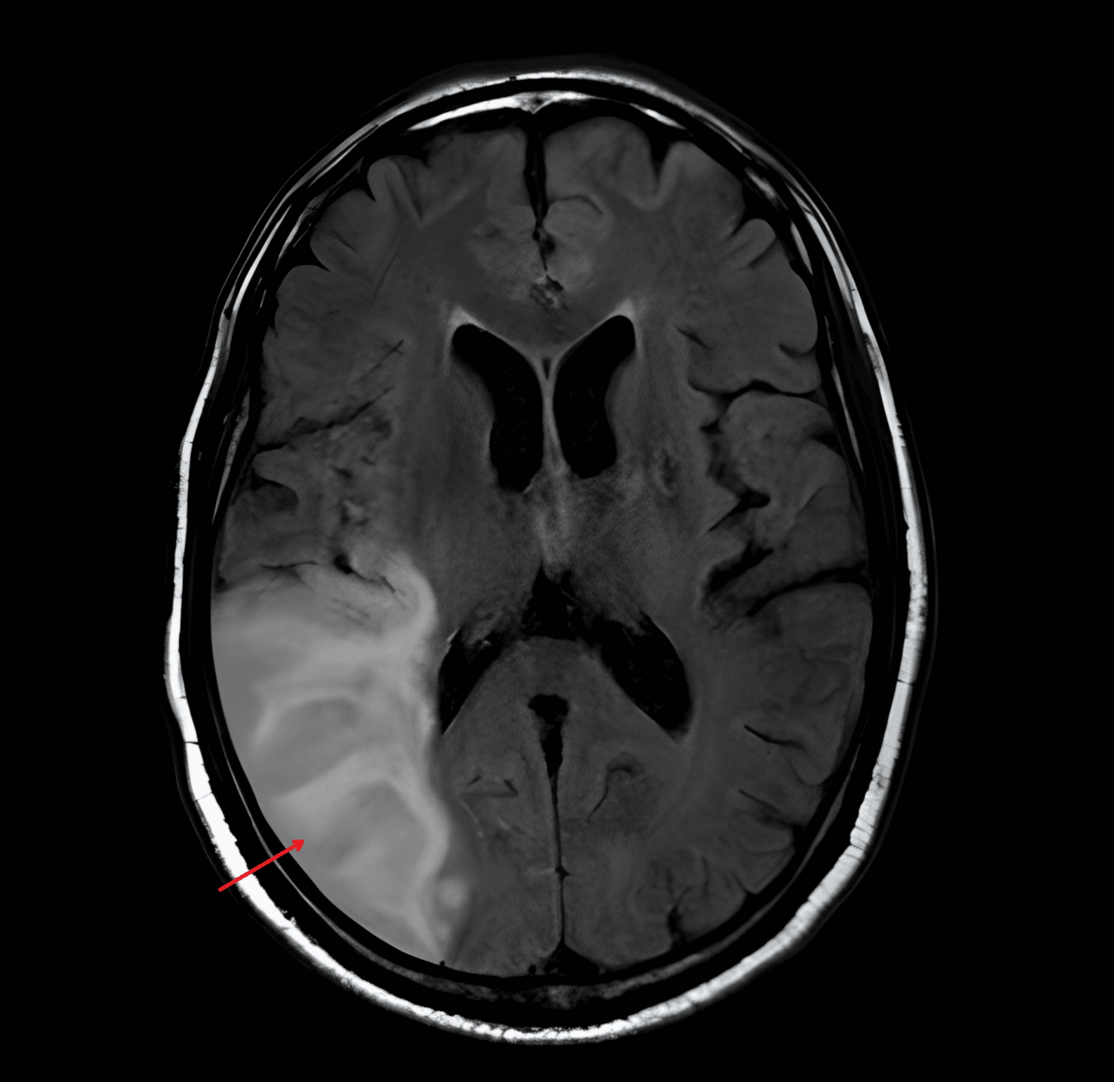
Axial image of the brain (T1-weighted) showing the right parietal cortical-subcortical hypodensity (red arrow). The magnetic resonance image confirmed the ischemic stroke in the territory of the middle cerebral artery.
